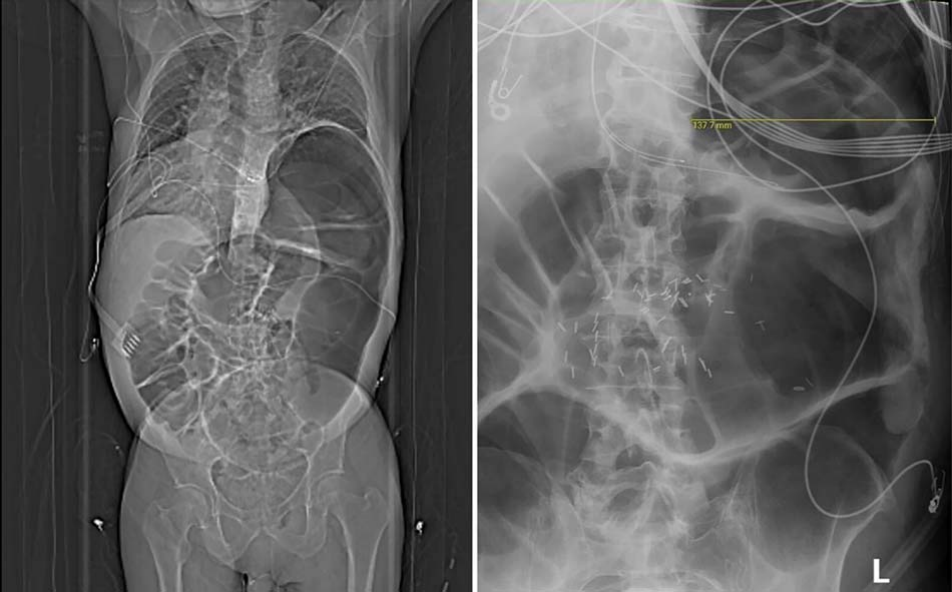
Scout CT image showing severe colonic dilation causing cranial displacement of the left hemidiaphragm and unilateral compression of the left thorax; abdominal radiograph after the second administration of neostigmine showing persistent colonic dilation measuring 13.8 cm.
